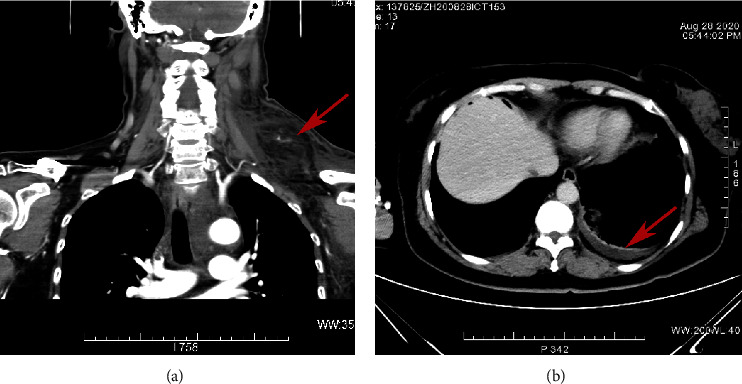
A computed tomography scan reveals suspicious neck cellulitis (a) and left pleural effusion (b).
